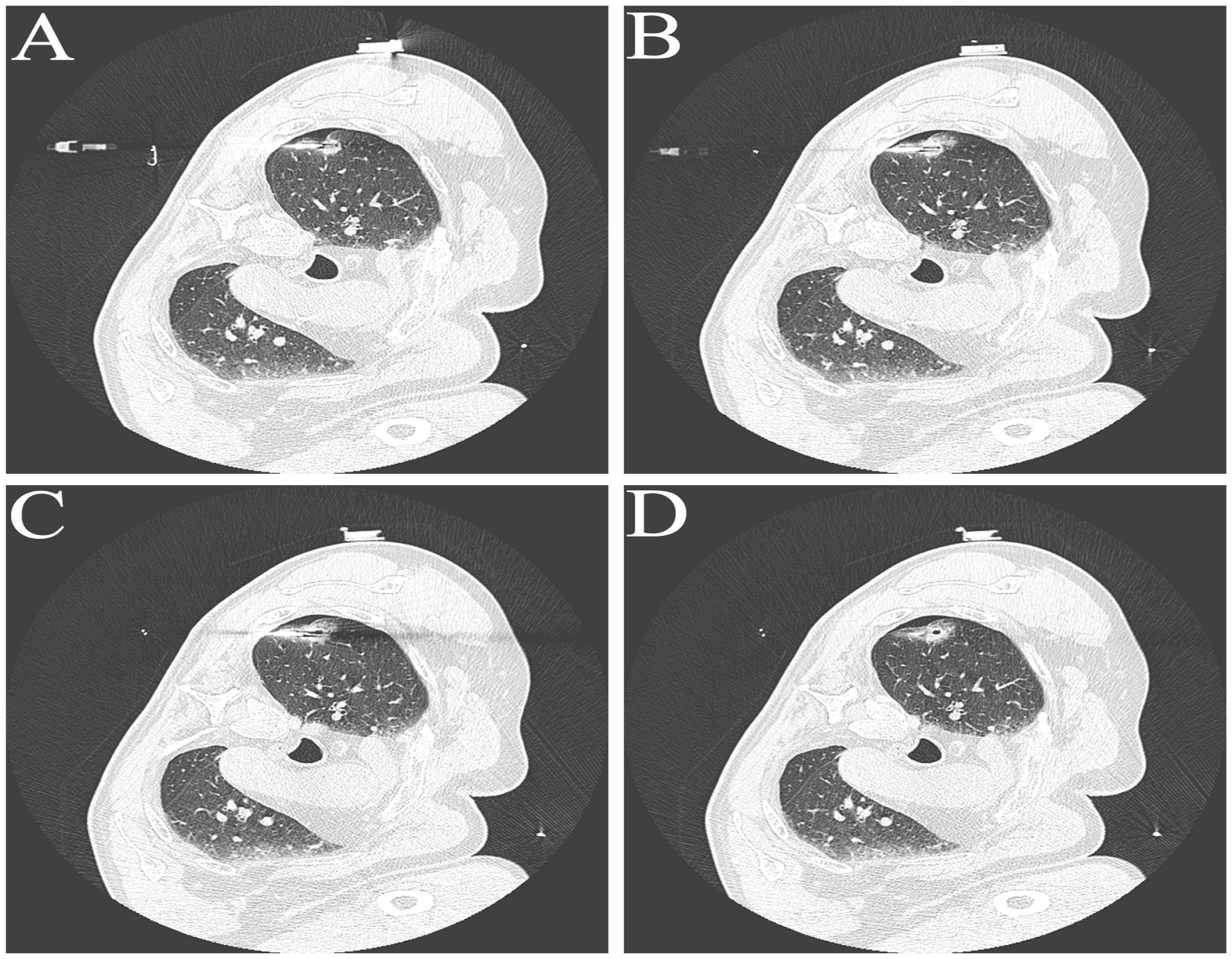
Representative Images in Laser Ablation Process. (A-C) Representative images of fiber optic positioning within tumor tissue during the ablation process. (D) Representative CT image of the tumor site after laser ablation.
